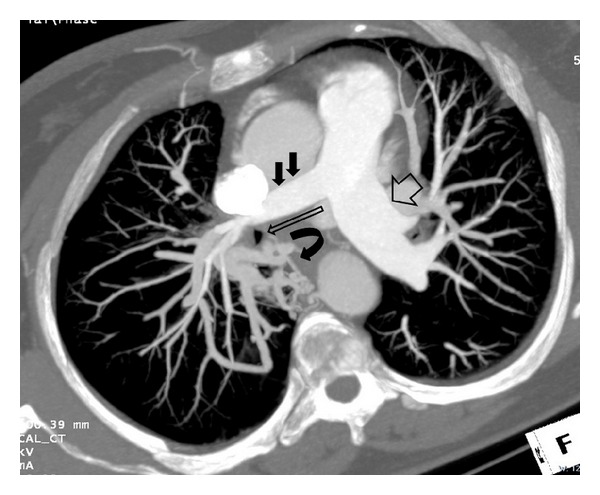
On Axial MIP CT image; at the upper and lower branches of the right main pulmonary artery (black arrows) diffuse hypoplasia, left main pulmonary artery (open thick arrow), hypoplasia of the right intermediate and middle lobe bronchus (open long arrow) was detected.
